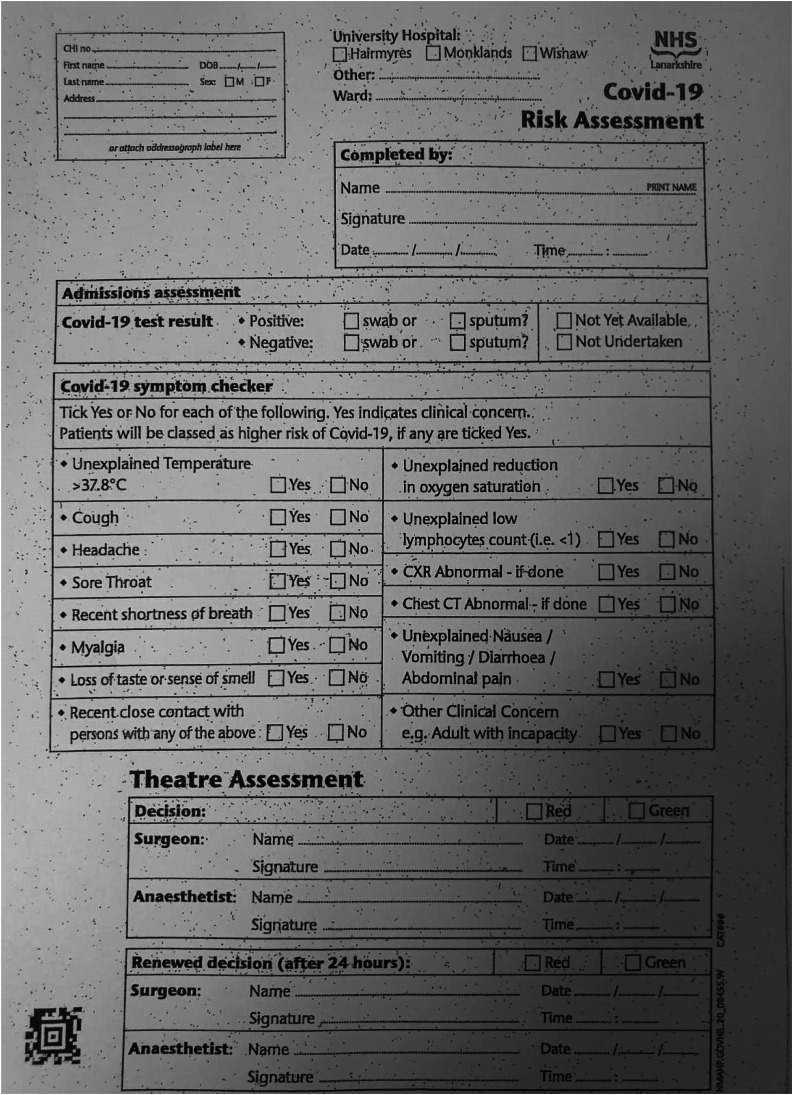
NHS Lanarkshire coronavirus disease 2019 (Covid-19) operating theatre risk assessment form. CXR = chest X-ray; CT = computed tomography
